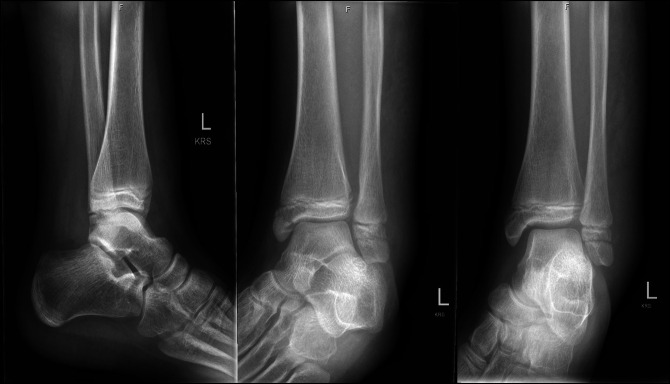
AP, mortise, and lateral view ankle radiographs of Case 3 after their injury, demonstrating a nondisplaced Salter-Harris type III medial distal tibia fracture and Ogden Type VII distal fibula fracture.
